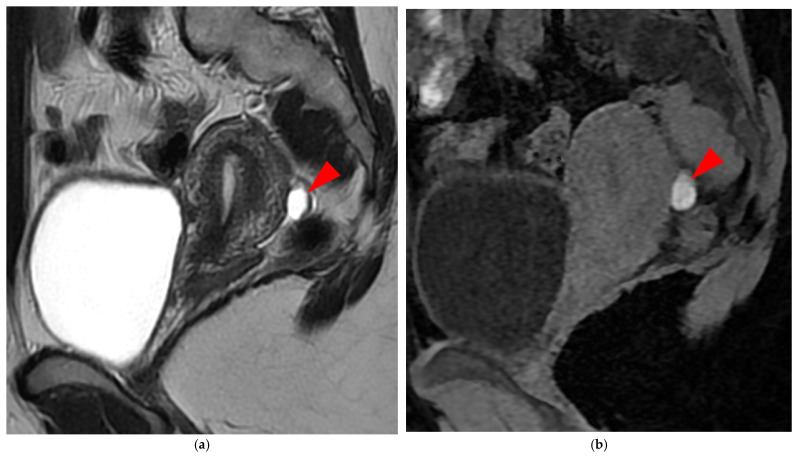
Pelvic MRI scan of a patient with superficial endometriosis. (a) Sagittal T2WI shows a hyperintense nodule located on the peritoneum of the pouch of Douglas (*arrowhead*). (b) Sagittal fat-suppressed T1WI reveals the hemorrhagic nature of this nodule due to its high signal intensity (*arrowhead*), allowing the diagnosis of superficial endometriosis.
